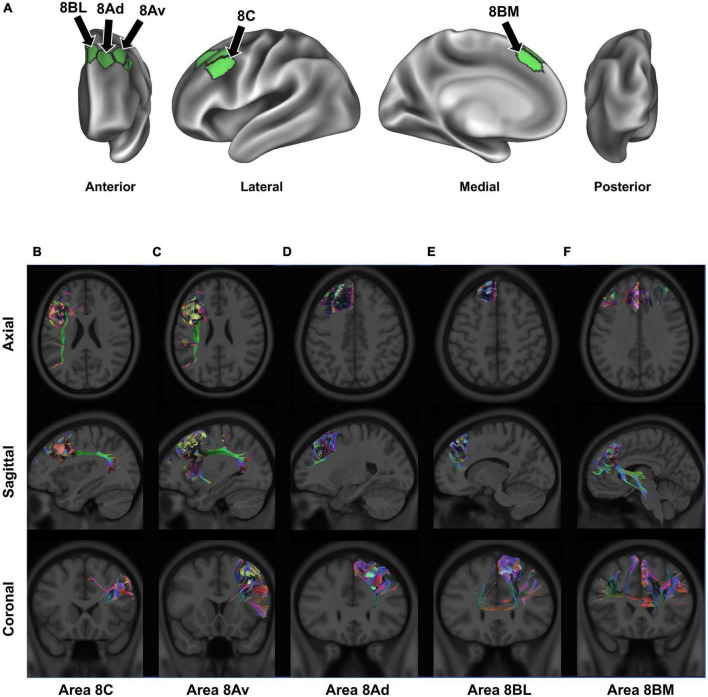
Connectivity of BA8. Panel (A) details the anatomical location of each BA8 subdivision, projected onto a left-hemispheric model brain. Panels (B–F) display the structural connectivity of all 5 area 8 subdivisions through tractography, projected onto a sample MRI image: area 8C (B), area 8Av (C), area 8Ad (D), area 8BL (E), and area 8BM (F). Note that there may be parallax error within the projections, given the two-dimensional nature of the images.
